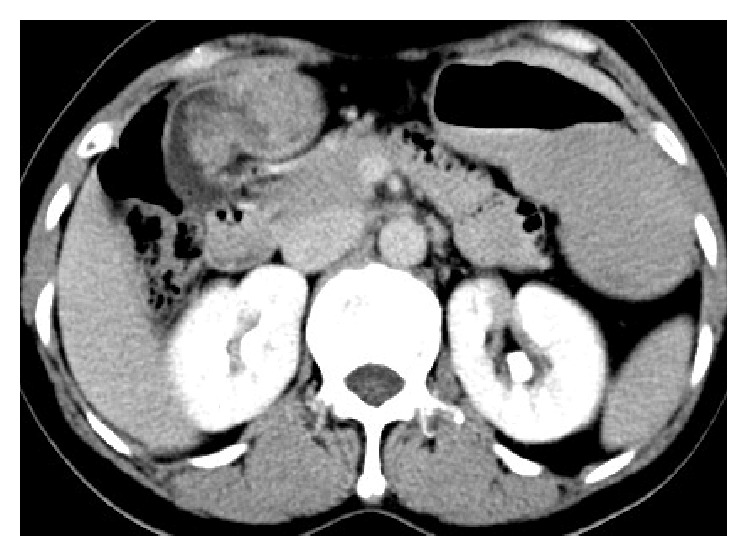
CT scan reveals a thickening gallbladder wall and heterogeneous enhancement of the gallbladder wall, with the interface between the gallbladder wall and the liver not apparent.
